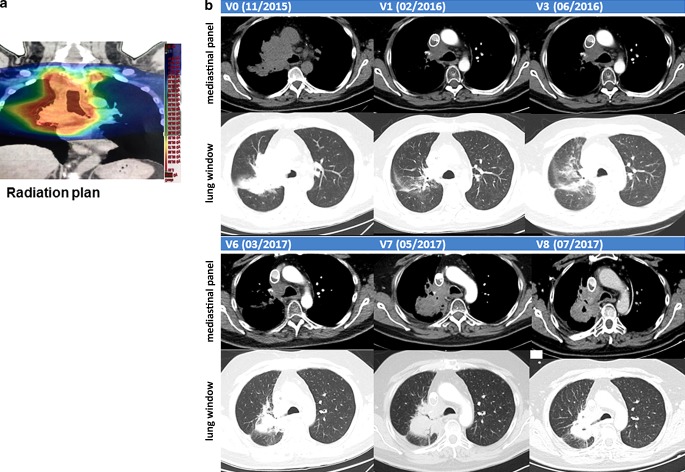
a Radiation plan. b Representative computed tomograpgy (CT) scans (upper panel: mediastinal window; lower panel: lung window) before and after therapies (V0–V8): lung at diagnosis (V0, 11/2015), after RCT (V1, 2/2016), after NK cell therapy (V3, 3/2016), after pseudo-tumor progress (V6, 3/2017), after 3 injections of nivolumab treatment (V7, 5/2017), and 20 months after diagnosis without tumor progression (V8, 7/2017)
